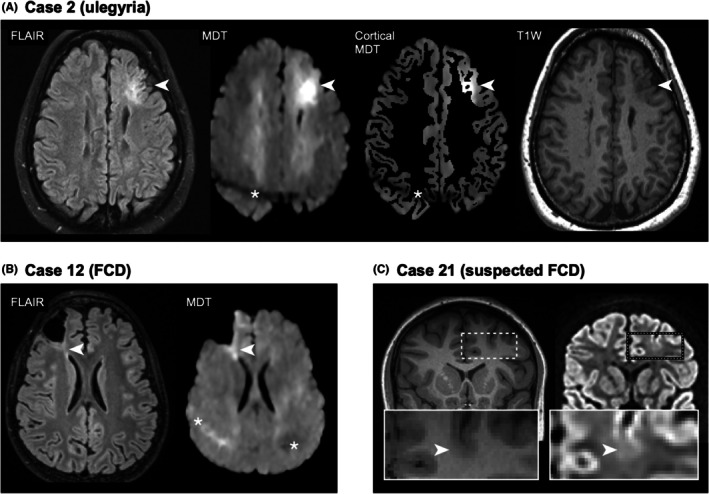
Ulegyria and focal cortical dysplasias (FCD). Panel A shows case 2 with ulegyria in the left frontal lobe. Hyperintensity is seen on both FLAIR and MDT involving the cortex and subcortex (white arrowheads). Cortical MDT confirmed cortical origin, and T1W showed apparent cortical thickening. An asterisk marks fat artifacts. Panel B shows case 12, a previously resected FCD. A transmantle sign in the right frontal lobe is visible on both FLAIR and MDT (white arrowheads). The overlying cortex had been resected, leaving no evident cortical component, though a mild signal increase was observed at the resection margin. Asterisks mark fat artifacts. Panel C shows case 21 with a bottom‐of‐sulcus dysplasia in the left frontal lobe (white arrowheads). The lesion was positive on FLAIR but negative on MDT. Cortical disruption was appreciable on high *b*‐value DWI (spherical encoding, *b* = 2500 s/mm^2^, white arrowheads), and cortical thickening was confirmed on T1W.
